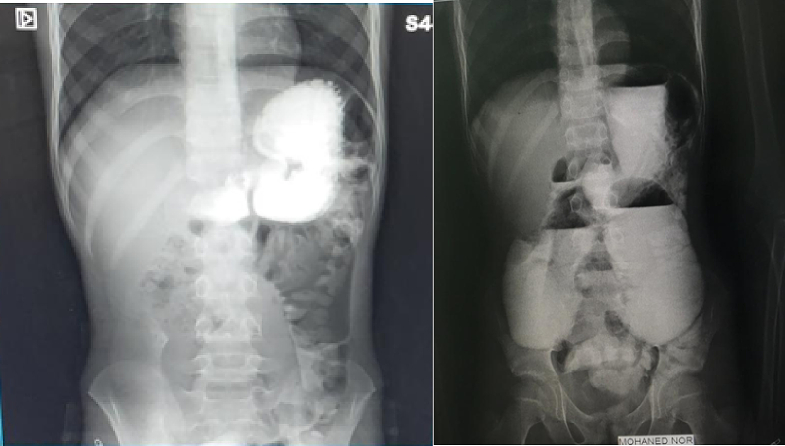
Post-contrast CT abdomen and pelvis revealed marked dilatation of the stomach, pylorus, and duodenum secondary to a short, tightly stenotic segment seen mostly at the duodenum-jejunal junction.
